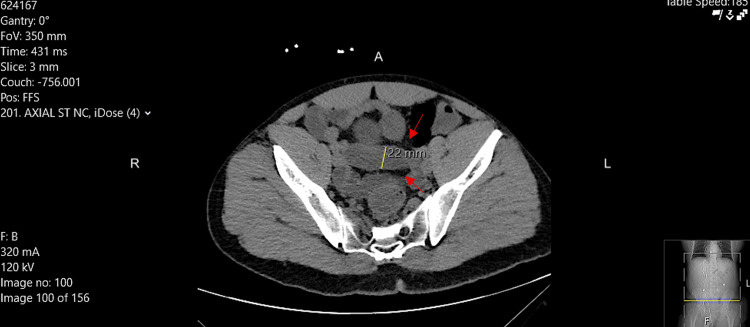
Axial Non-Contrast CT of the Abdomen and Pelvis Showing Colonic Inflammation Axial non-contrast CT of the abdomen and pelvis demonstrating segmental colonic wall thickening with mild pericolonic inflammatory changes (red arrows). The yellow line represents the measured colonic lumen diameter of 22 mm.
